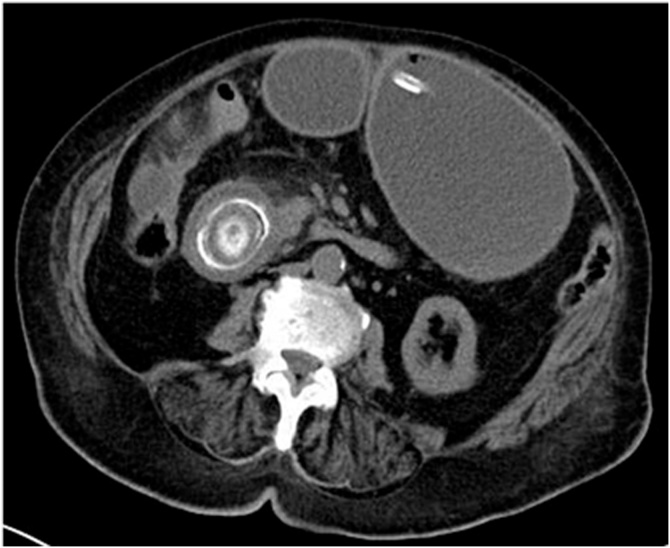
Abdominal CT scan showing a dilated stomach and a calcified stone impacted in the second portion of duodenum.
